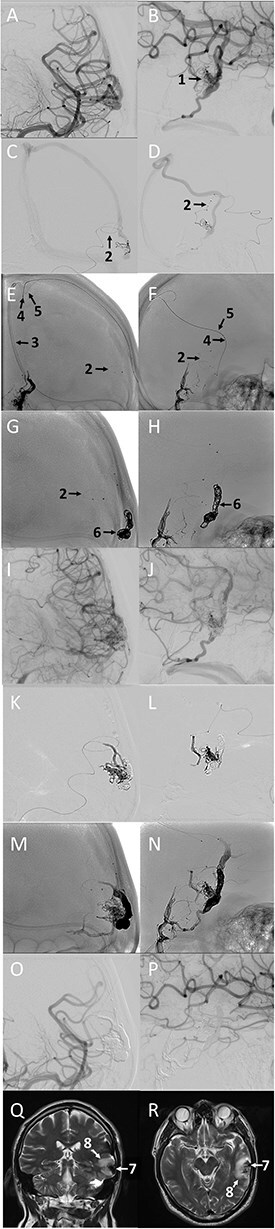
Third stage - total embolization of left temporal lobe AVM from combined transarterial and transvenous approach. A, B - DSA from the left ICA in frontal (A) and lateral (B) views; C, D - DSA from the left middle cerebral artery afferent, frontal (C) and lateral (D) views; E,F - craniograms showing the tools placement before embolization, frontal (E) and lateral (F) views; G, H - craniograms showing the complex of coils in the venous drainage of AVM, frontal (G) and lateral (H) views; I, J - DSA from the left ICA showing AVM contrasting after positioning of coils in the venous drainage, frontal (I) and lateral (J) views; K,L - transarterial embolization with non-adhesive embolic agent - Squid 18 (Balt), frontal (K) and lateral (L) views; M, N - craniograms showing carst of non-adhesive composition and coil’s complex after transvenous stage of AVM embolization with embolic agent - Squid 18 (Balt), transvenous ‘pressure coocking’ technique, frontal (M) and lateral (N) views; O, P - DSA from left ICA after total embolization, frontal (O) and lateral (P) views; Q, R - postoperative brain MRI showing postoperative edema around the total embolized AVM of the left temporal lobe, coronal T2 weighted (Q) and axial T2 weighted MRI (R) views. Arrows: 1 - nidus of the left temporal lobe AVM; 2 - afferent from the left middle cerebral artery; 3 – Guide catheter in the superior sagittal sinus; 4 - sonic 1.5 F 45 (Balt) microcatheter placed in the AVM draining vein; 5 - headway 17 microcatheter (Microvention) positioned in the draining vein; 6 - coil complex placed in the AVM draining vein; 7 - embolized AVM of the left temporal lobe; 8 - perifocal edema around the total embolized AVM.
